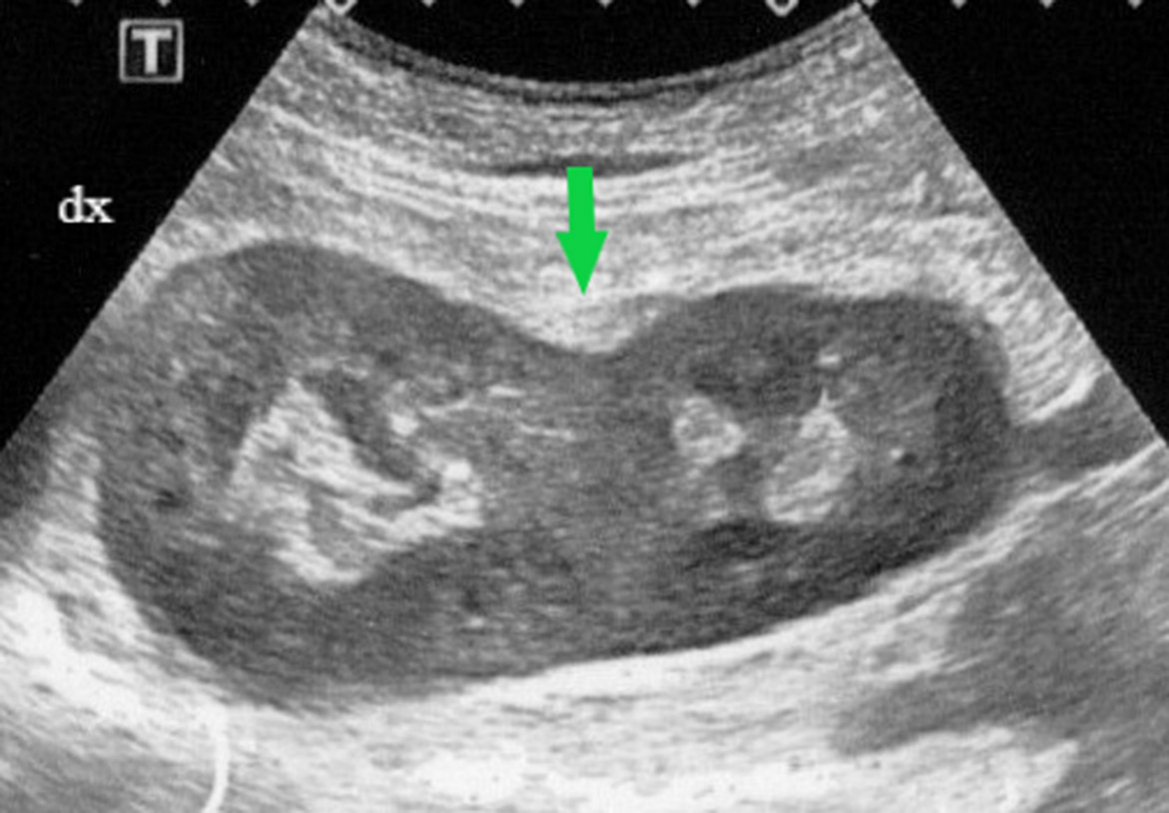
Ultrasound image (axial view): fusion of kidneys at medial parenchymal
